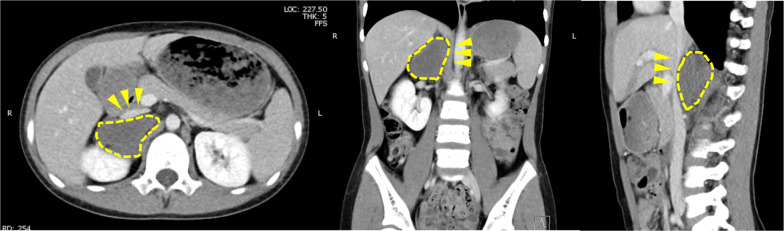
Abdominal CT showed that retroperitoneal tumor (dotted line) compressed IVC (arrowhead) and duodenum
